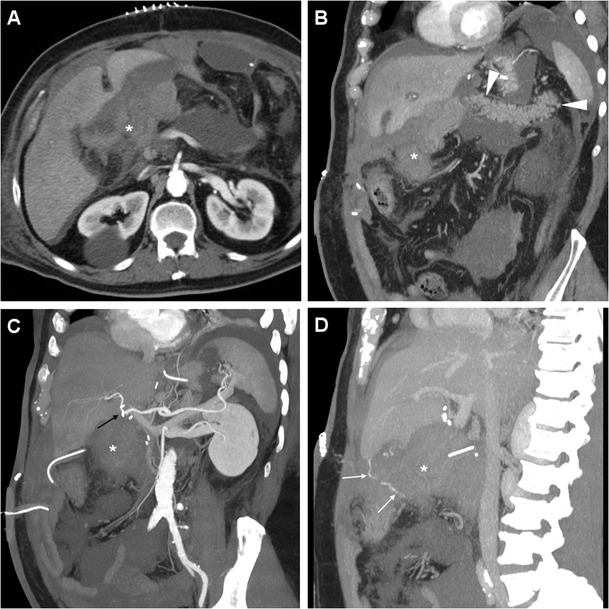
Venous extraluminal haemorrhage in a 74-year-old man following pylorus-preserving PD for pT3N1 CBD adenocarcinoma, suffering from hypotension, abdominal pain and blood from drainage tube on postoperative day 7. Urgent CT showed haemoperitoneum, fresh blood (*) extending from the surgical bed in the subhepatic space and mesentery, normal appearance of RP (*arrowheads* in b). CT-angiography MIP reconstructions (c) did not detect active arterial bleeding or pseudoaneurysms, particularly at the gastroduodenal artery “stump” (*thin black arrow*). In the venous phase (d) serpiginous CM extravasation (*thin arrows*) was detected. Emergency surgery was required to control oozing venous bleeding at the transverse mesocolon. (Partially adapted from Tonolini [38])
